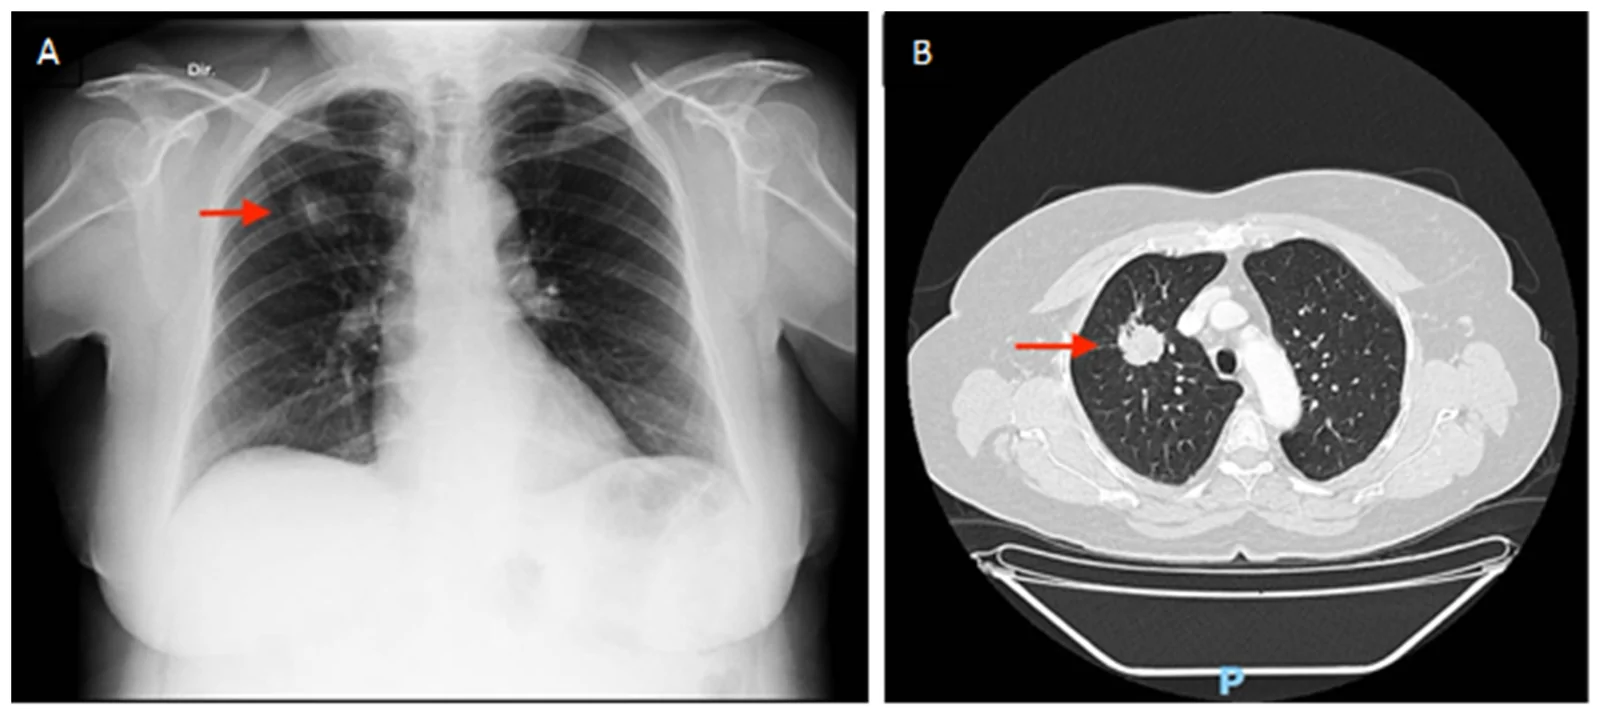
Initial chest imaging demonstrating the right upper lobe pulmonary nodule. Red arrows indicate the pulmonary lesion. (A) Chest radiograph showing the pulmonary lesion in the right upper lung field. (B) Axial chest computed tomography demonstrating a lobulated 2.9 cm solid nodule in the right upper lobe.
